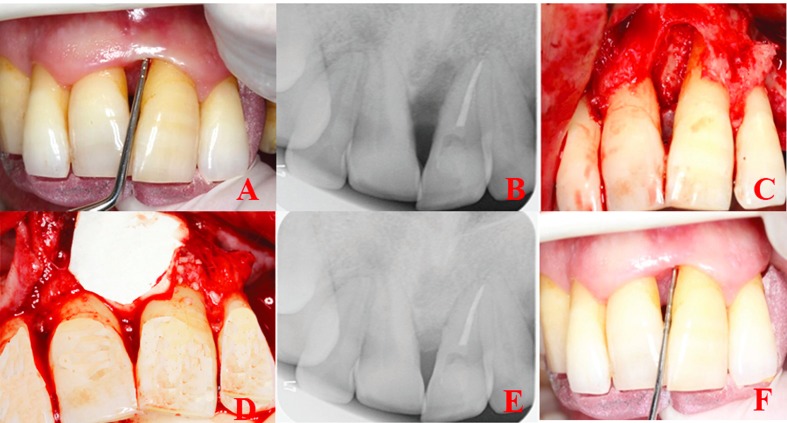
GTR procedure in the treatment of an intrabony defect with endo-perio lesion. A) Preoperative clinical view, B) Baseline radiograph, C) Intraoperative clinical image of typical intrabony defect, D) The defect is filled with allograft and covered with a resorbable membrane, E) Nine months postoperative radiograph, F) Nine months of post-operative clinical view.
